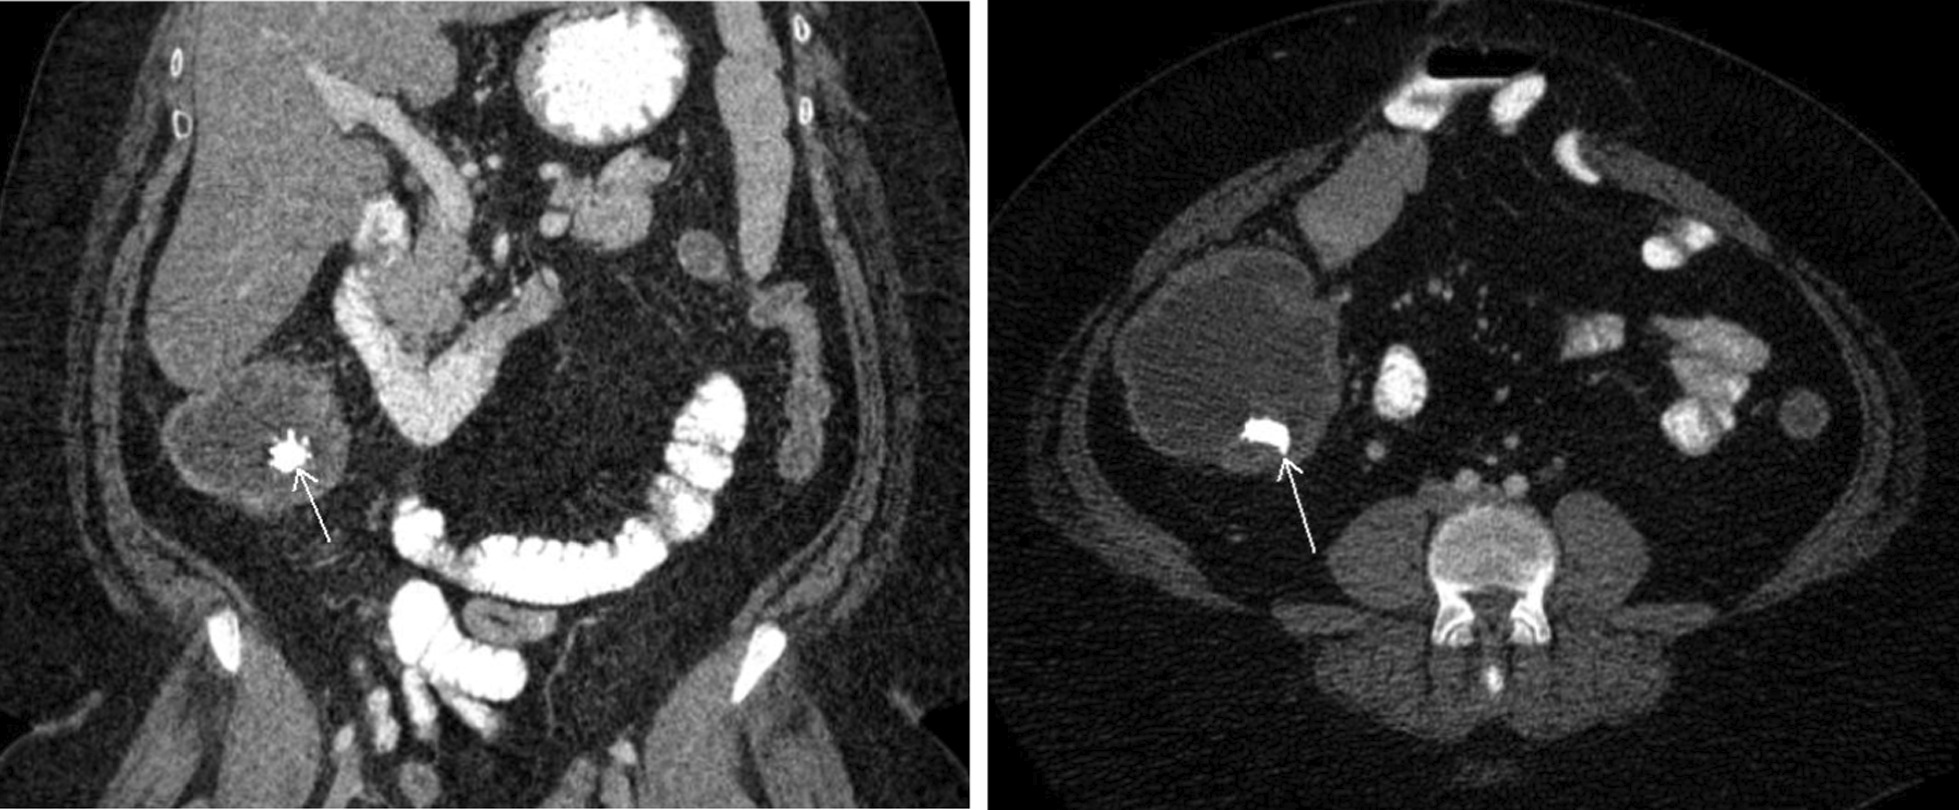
CT of the abdomen and pelvis with contrast. CT scan of the abdomen and pelvis showing postsurgical changes of radical cystectomy with ileal diversion in the right lower quadrant. Ileolithiasis is present within the Indiana pouch (arrow). There is no hydroureteronephrosis. No other acute abnormality is present in the abdomen or pelvis
